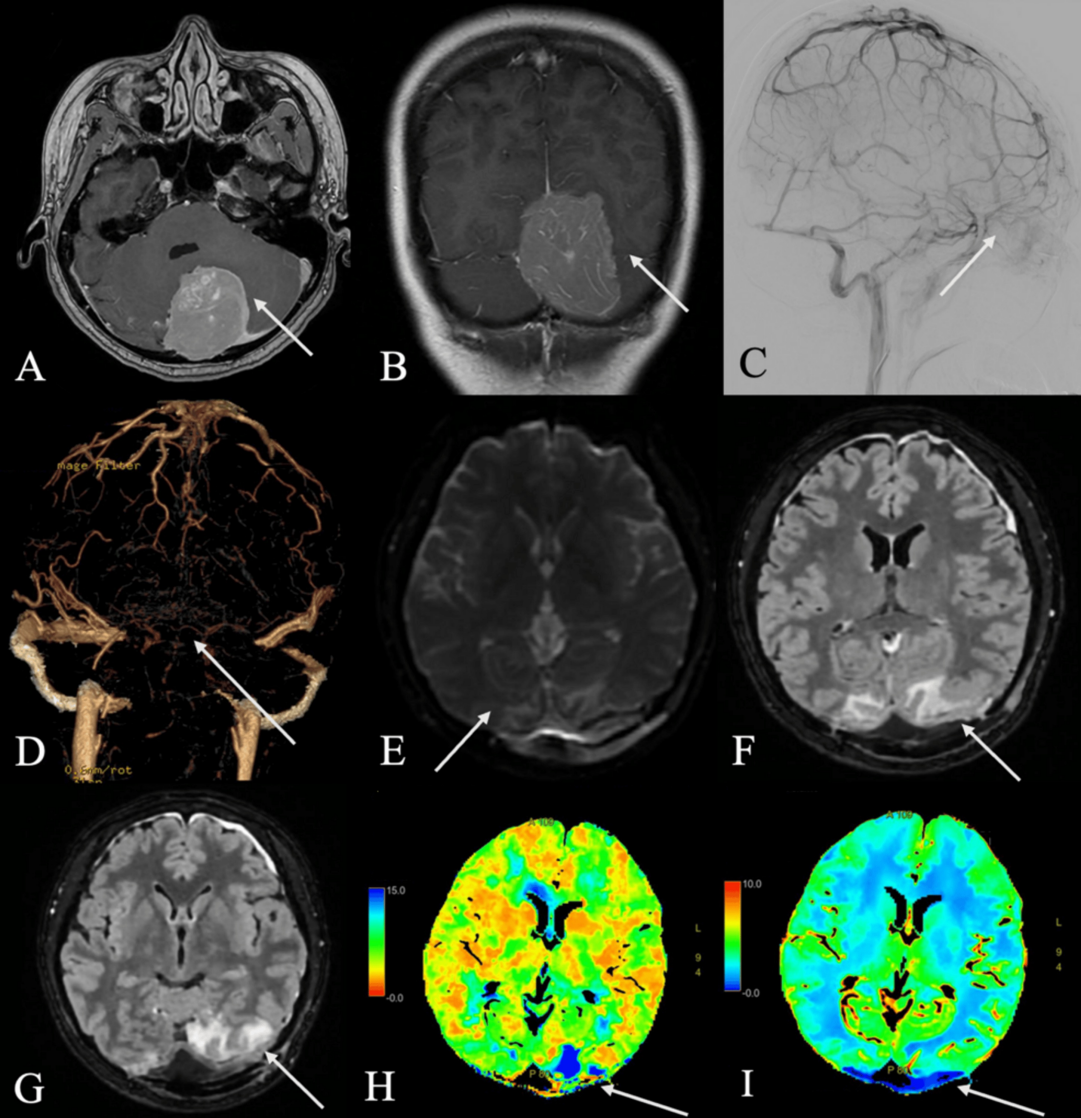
Key Images for Patient 1 Preoperative investigations characterizing lesion: (A) Axial post-contrast T1-weighted MRI demonstrating a large left torcular mass; (B) Coronal post-contrast T1-weighted MRI demonstrating supra- and infratentorial extension; (C) Cerebral angiogram demonstrating absence of opacification of the torcula, posterior SSS, and bilateral TS. Postoperative investigations: (D) CT venogram reconstruction demonstrating filling defect and thrombosis of the straight sinus; (E) Axial diffusion-weighted MRI demonstrating no infarction in the bilateral occipital lobes; (F) Axial FLAIR MRI demonstrating bilateral occipital lobe edema; (G) Axial FLAIR MRI demonstrating extension of edema to the left parietal lobe; (H) Axial CT perfusion demonstrating prolonged mean transit time, particularly in the left occipital lobe; (I) CT perfusion demonstrating normal cerebral blood volume, indicating venous stasis. MRI, magnetic resonance imaging; CT, computed tomography; SSS, superior sagittal sinus; TS, transverse sinus; FLAIR, fluid-attenuated inversion recovery
